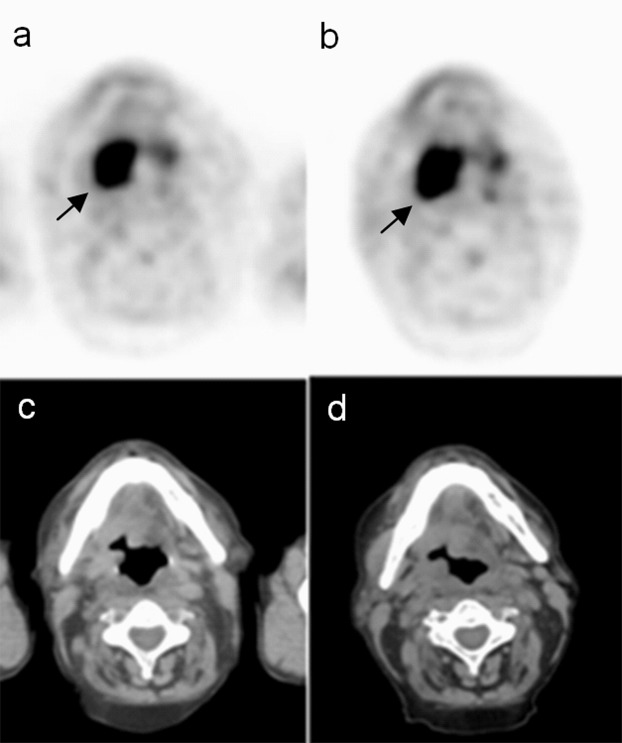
The malignant lesion within the pharynx showing increasing [18 F]FDG uptake over time. (a) - PET initial scans; (b) - PET delayed scans; (c) - CT initial scans; (d) - CT delayed scans; black arrows show the malignant mass infiltrating oropharynx.
